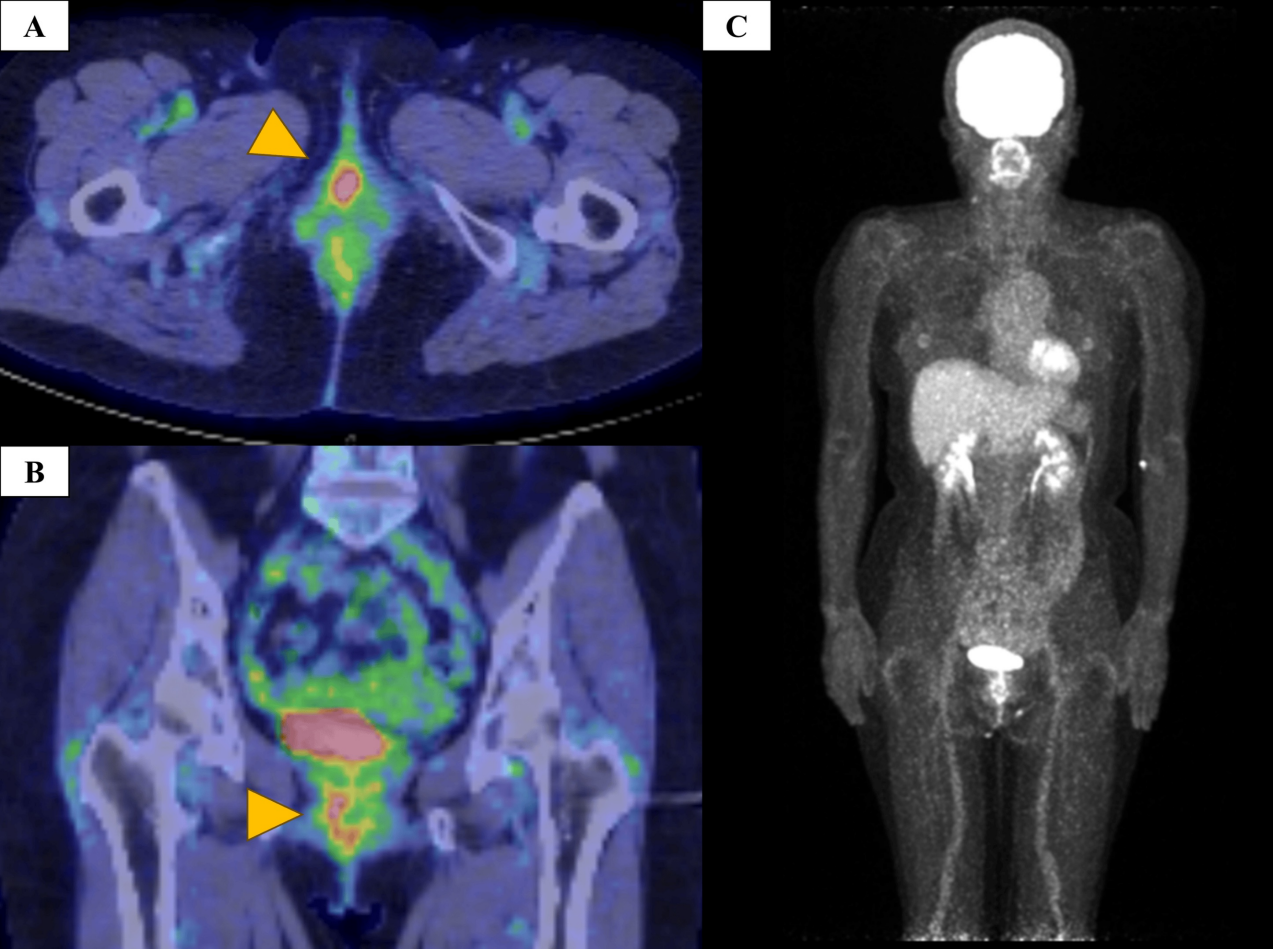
Pre-treatment FDG-PET/CT (A, B) Axial and coronal imaging showing FDG uptake in the vagina and urethra (arrowheads). (C) MIP image showing no lymph node metastasis or distant metastasis. FDG: ^18^fluorodeoxyglucose; PET/CT: positron emission tomography/computed tomography; MIP: maximum intensity projection
